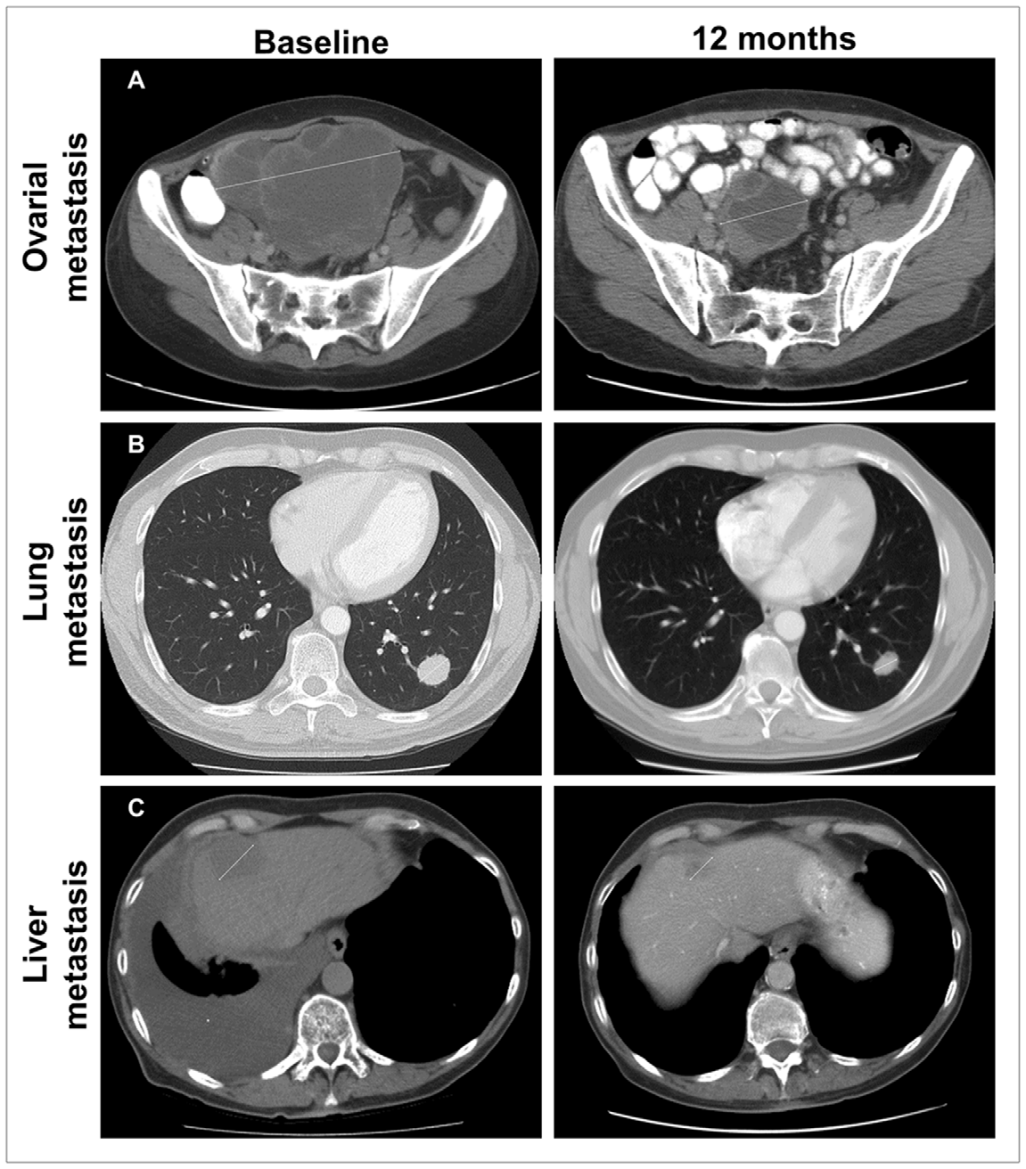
Computed tomography showing partial responses in three different patients at baseline and at 12 months. Panel A shows ovarian metastases in a 43 years old woman. Panel B shows lung metastases in a 50 years old man. Panel C shows liver metastases and pleural effusion (*) in a 70 years old man. Arrows show the largest diameter of the lesions.
